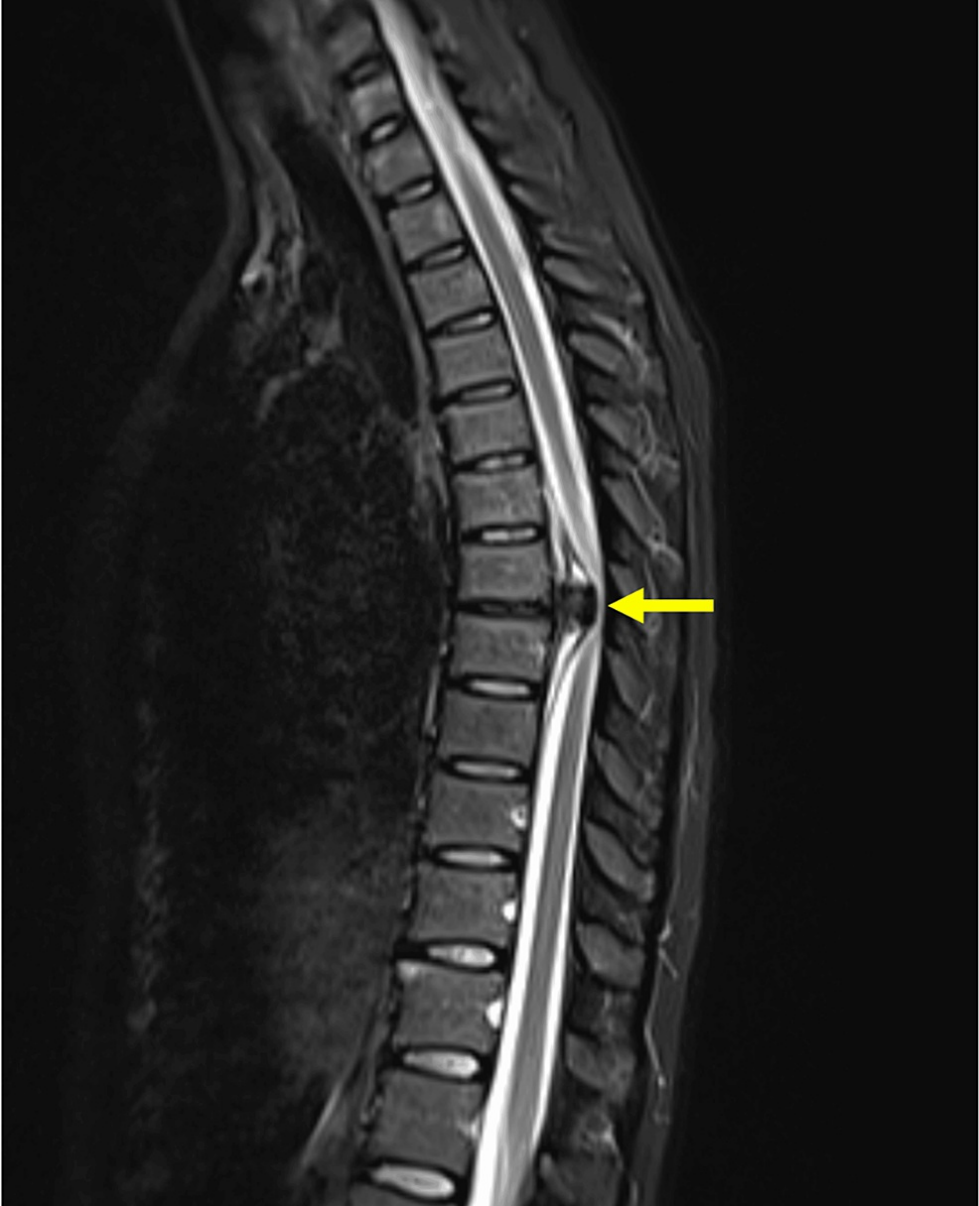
MRI sagittal image showing herniated lesion. Arrow: herniated disc.
